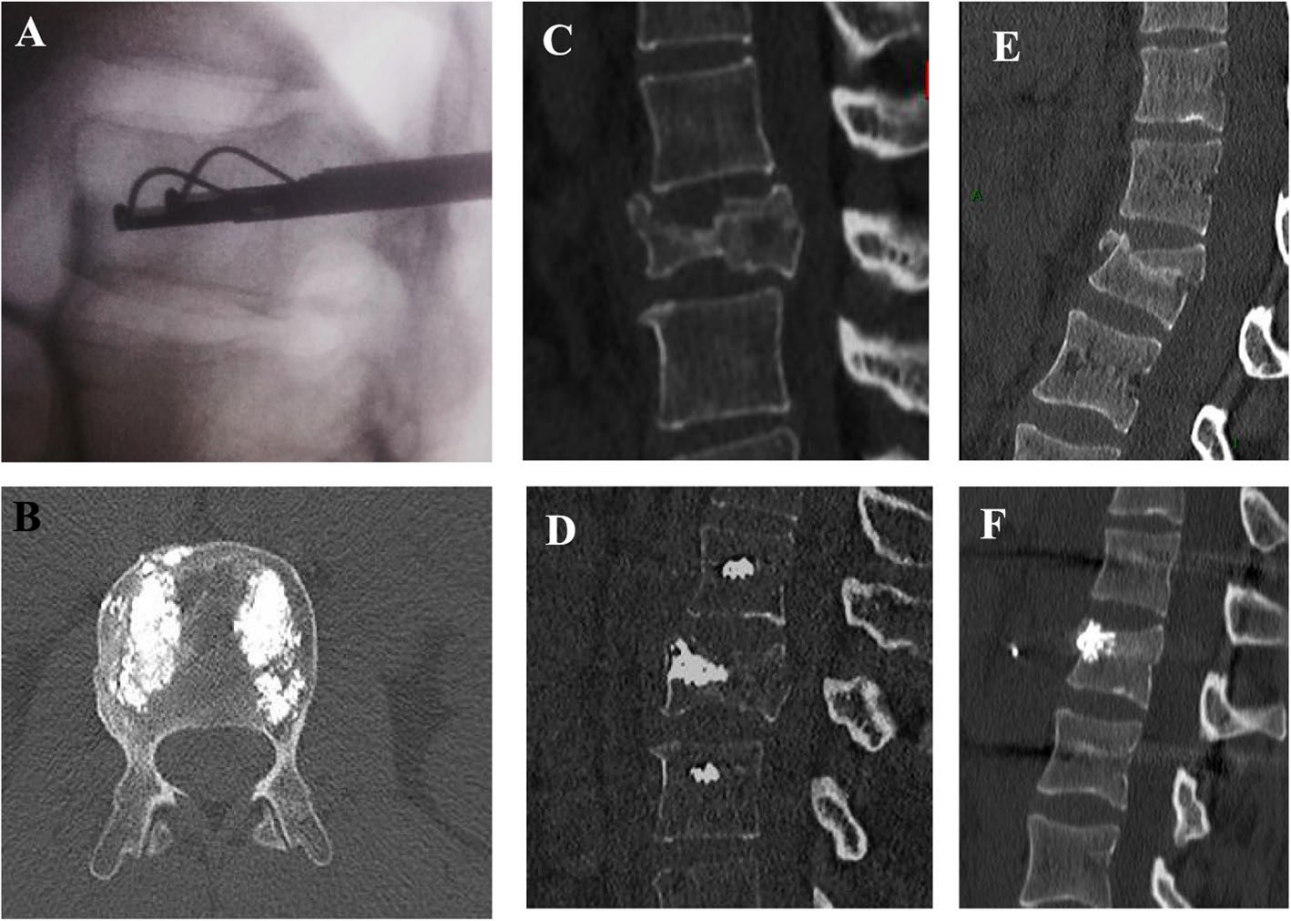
A: Per-operative C-arm imaging of the reduction of the superior endplate by the flexible lamella. B: Axial CT-scan view of vertebral body cementing. C and D: Same patient, pre- and postoperative sagittal CT-scan imaging after a vertebroplasty by Tektona™. E and F: Same patient, pre- and postoperative sagittal CT-scan imaging after a vertebroplasty by Tektona™
